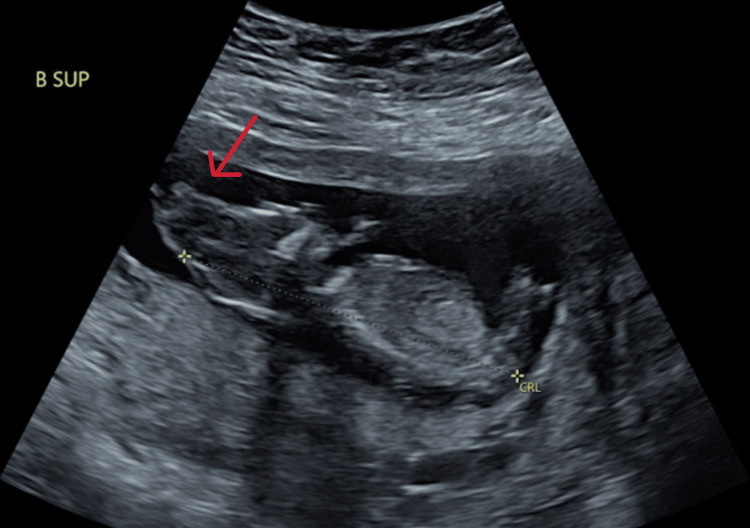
Ultrasound of twin B at 13.1 weeks gestation demonstrating exencephaly Red arrow is pointing to the cranium.
